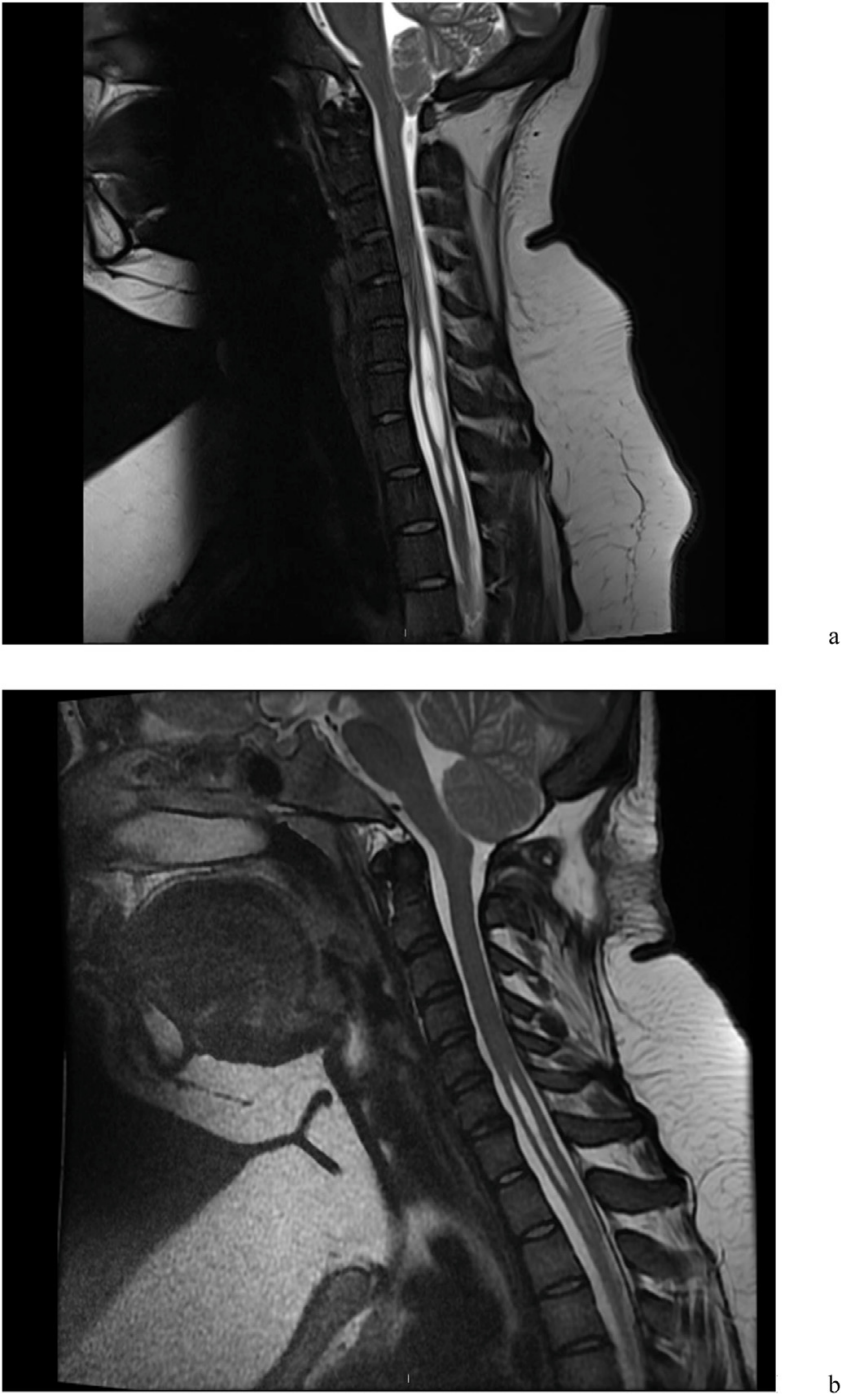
Different causes of tonsillar descent. I. Small posterior fossa. Sagittal T2 weighted C-spine MRI of a patient pre (a) and post (b) posterior fossa decompression with a patch due, showing the early reduction in syrinx size following enlargement of the posterior fossa, and tonsillar cautery.
